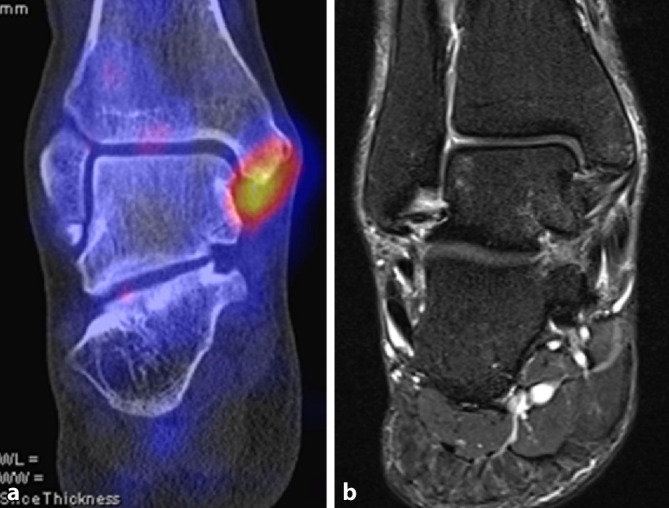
Ha et al. verglichen erstmals SPECT/CT systematisch mit MRT. Bei 50 Patienten mit 147 Läsionen an Knochen, Gelenken, Ligamenten und Sehnen wurden die Aussagen beider Verfahren analysiert. Bei Knochenläsionen und Ligament‑/Sehnenpathologien war die Spezifität des SPECT/CT mit 57 % vs. 10 % bzw. 73 % vs. 8 %, signifikant höher als die der MRT [9]. Eine andere Studie wies ebenfalls darauf hin, dass symptomatische Bandläsionen mit der SPECT/CT spezifisch nachgewiesen werden können [18]. Abb. 6 zeigt eine nur im SPECT/CT nachweisbare schmerzhafte Stressreaktion im Innenband (Deltaband) des oberen Sprunggelenkes.
